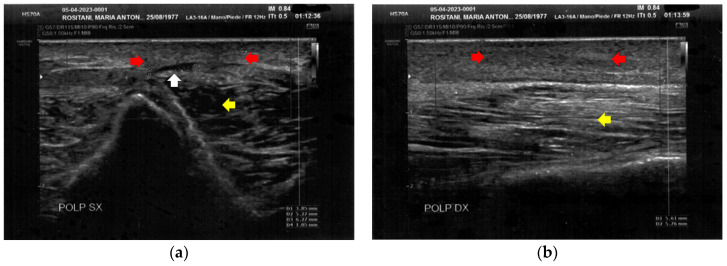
Ultrasonography of the calves at T0. (a) Cicatricial fibrosis (red arrows) is evident on the left calf on the superficial layers. Phlogosis extends to the deeper layers (yellow arrow). A small fluid element (white arrow) is observed at the muscular level; (b) Cicatricial fibrosis (red arrow) is also evident on the right side, where the muscular structure (yellow arrow) appears preserved.
